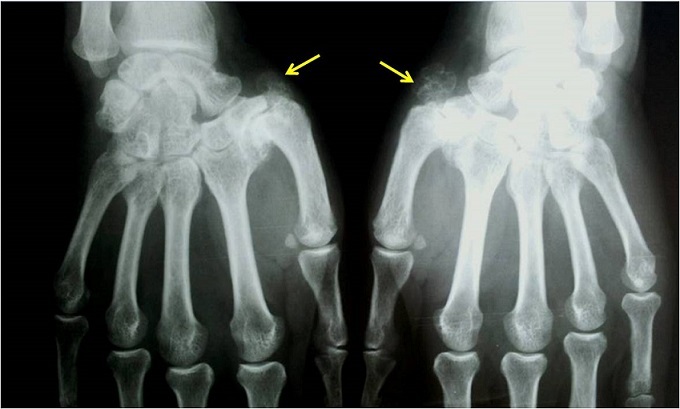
Radiographie standard des 2 mains: multiples formations de petites masses arrondies de tissu cartilagineux réalisant l'aspect en en chou-fleur
